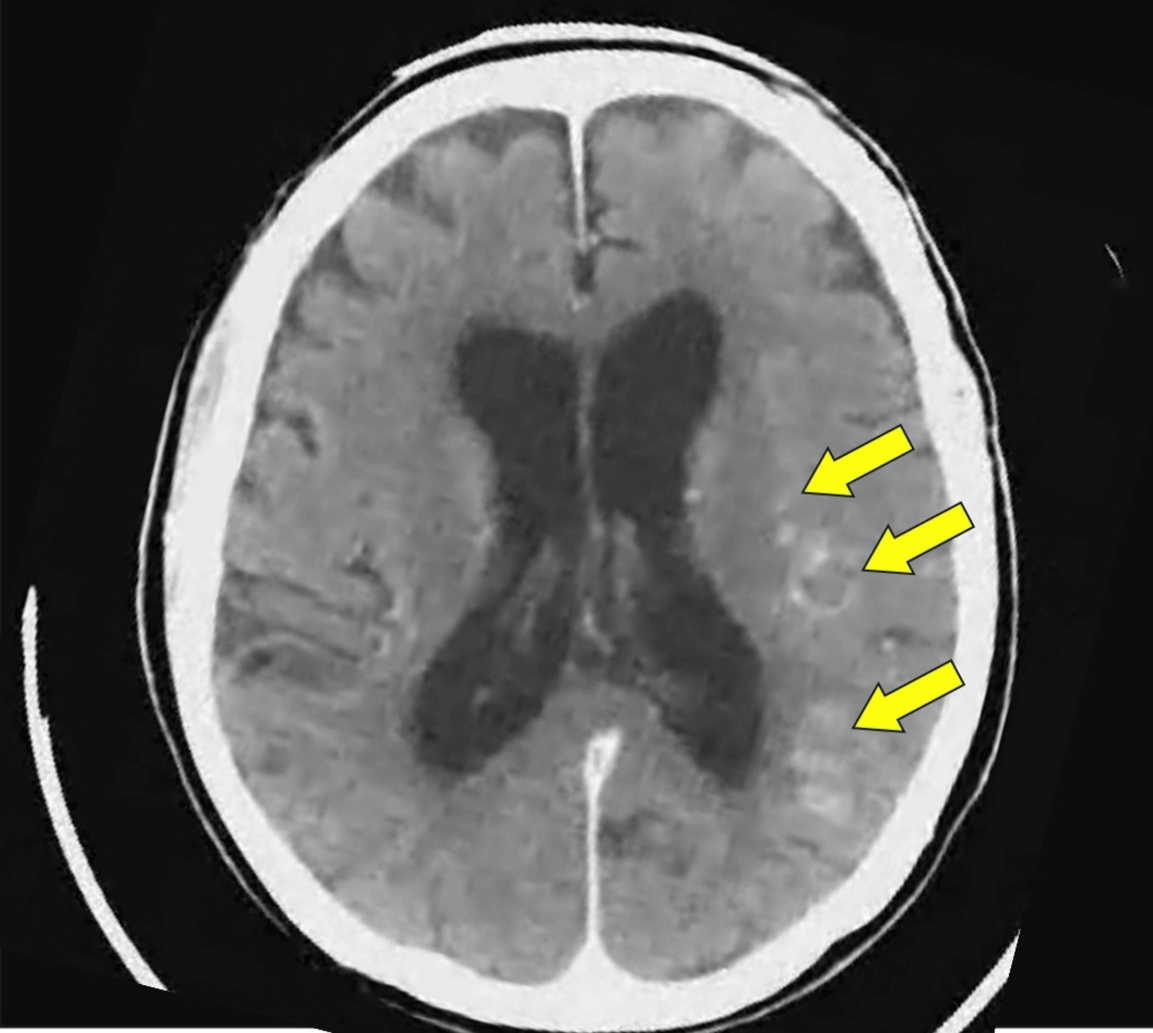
Computed tomography after reperfusion on the day of surgery A slight cerebral hemorrhage or contrast accumulation is observed in the ischemic region (arrows).
